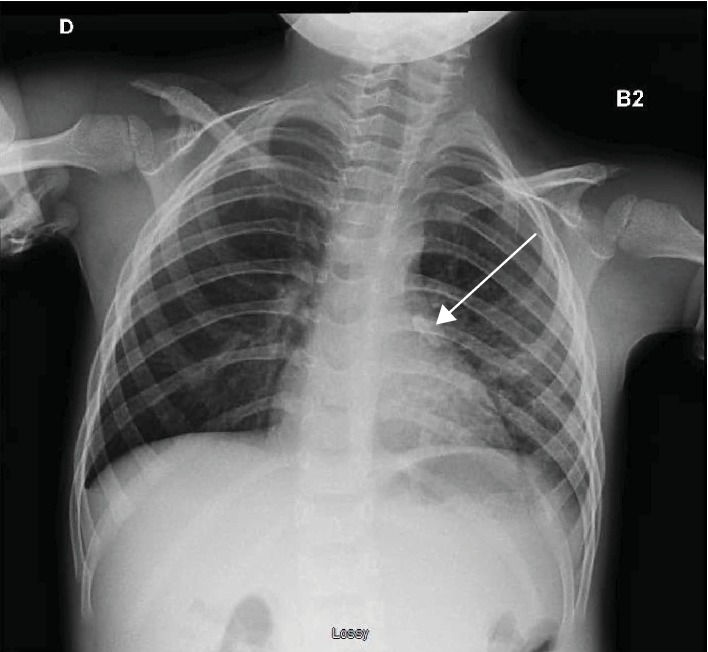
The lung X-ray performed three weeks after tooth extraction shows the tooth inside the left lung.
